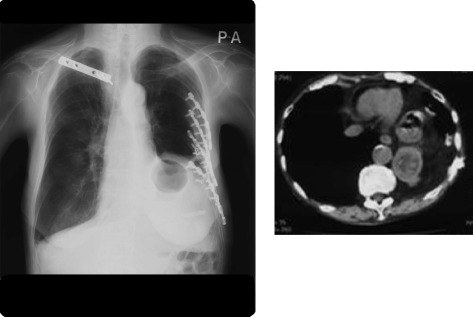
Chest X-ray and chest CT of case 1 Chest X-ray (left) and chest CT (right) show a left diaphragm hernia. The left diaphragm deviates upwards into the thoracic cavity and gastric gas is seen in the gastric body
